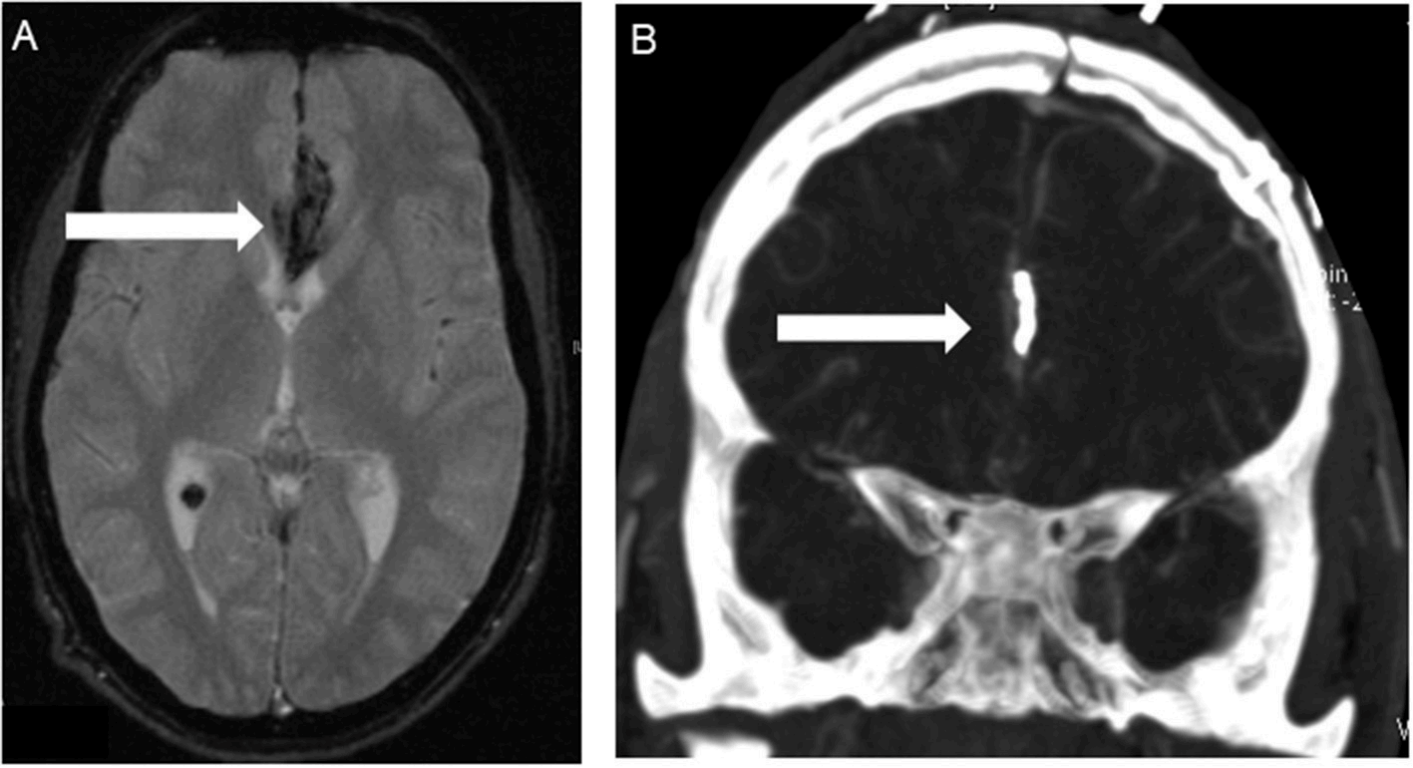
Patient No. 5 with subarachnoid hemorrhage because of ruptured left pericallosal artery aneurysm. (A) T2-wighted MRI scans showed SAH in the frontal interhemispheric region (arrow) due to the pericallosal artery aneurysm. (B) Postoperative computed tomography angiography (CTA) confirmed correct positioning of the clip (arrow) without any findings of vasospasm or ischemia.
